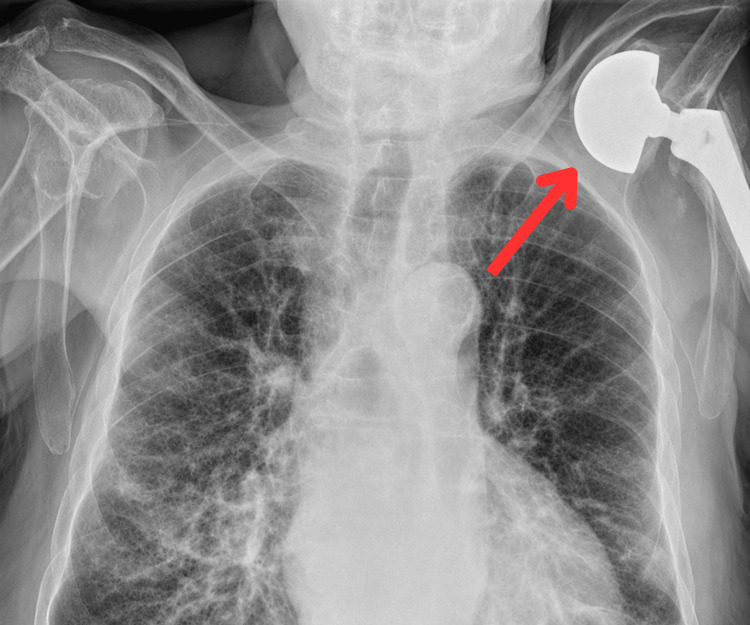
AP chest radiographs AP: anteroposterior
